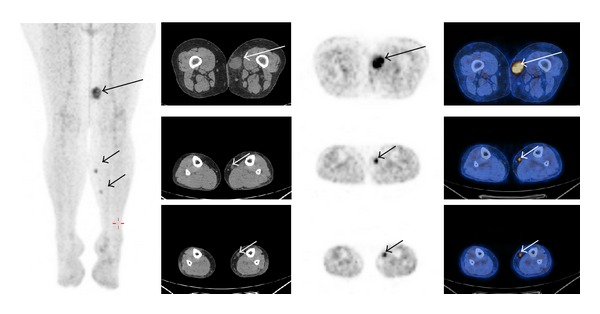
False negative a 35-year-old patient with a large round cell liposarcoma of the right ankle (top, small arrow) required nephrectomy for a large infrarenal mass (bottom, long arrow) which in retrospect was a non-FDG-avid lesion on the staging FDG-PET scan (middle, long arrow).
